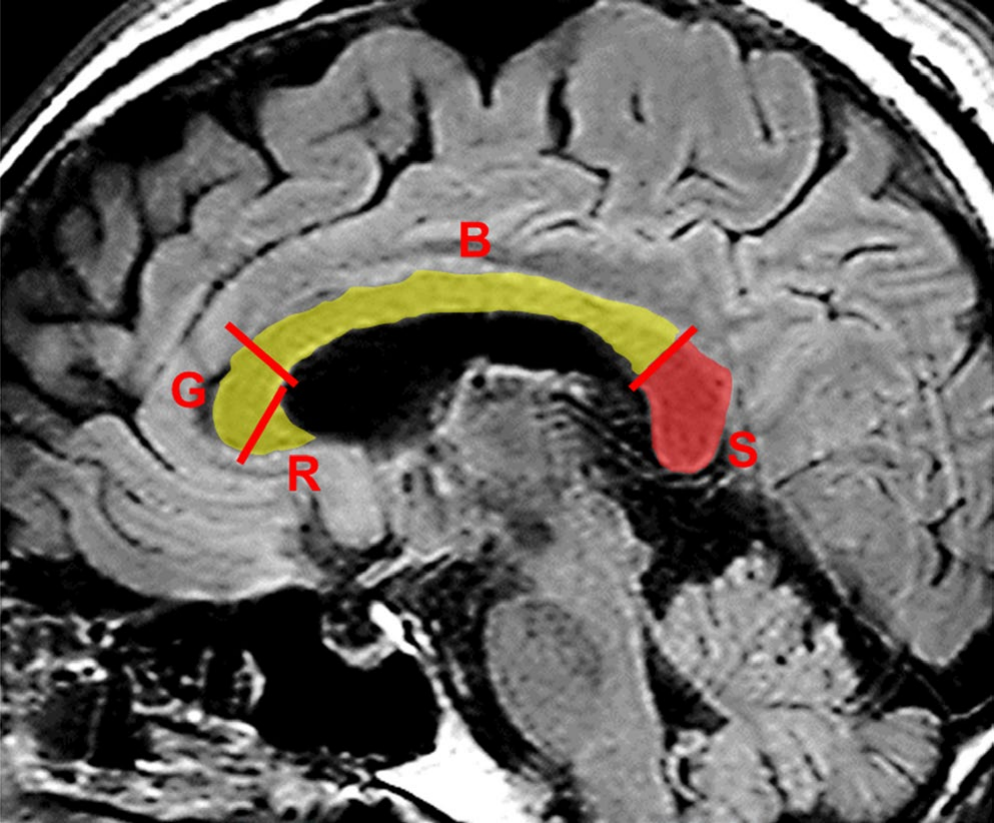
Midsagittal view of the splenium. Midsagittal fluid‐attenuated inversion recovery—weighted magnetic resonance image shows the corpus callosum and the splenium (S, in red). According to the conventional partitioning scheme, the splenium corresponds to the posterior 20% of the corpus callosum, which is separated by the border line perpendicular to the line linking the most anterior and posterior points of the corpus callosum. B, body; G, genu; R, rostrum
